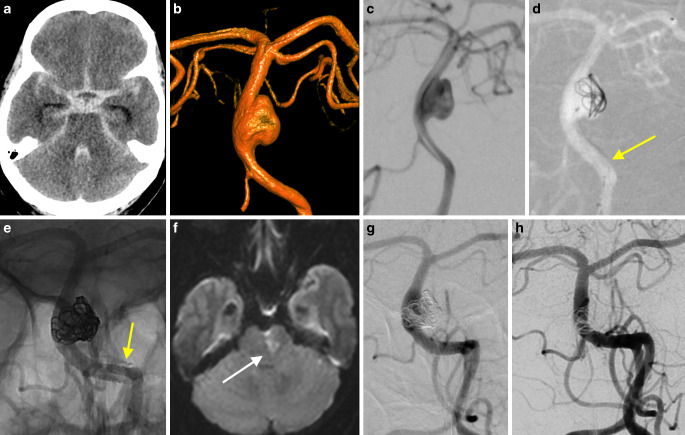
This 62-year-old female patient was admitted with diffuse SAH (a), Hunt and Hess grade 3 due to a wide-neck side-wall aneurysm of the basilar artery (b and c). The aneurysm was treated with partial coiling and flow division using the p64 flow modulation device in jailing technique (d and e). Right hemiparesis was noted after weaning and a corresponding left mediolateral pontine ischemia was confirmed on MRI (f). The emergently performed diagnostic angiography (g) showed no stent thrombosis. The most recent follow-up angiography performed 1 year after treatment shows complete exclusion of the aneurysm and patency of the stent (h). a axial NCCT, b 3D-VRT (Volume Rendering Technique), c working projection, d FDS-assisted partial coiling, the *arrow* points to the yet undetached FDS, e unsubstracted view of final run after partial filling of the aneurysm and detachment of the FDS (*arrow*), f axial DWI, g and h frontal views of left vertebral injections
